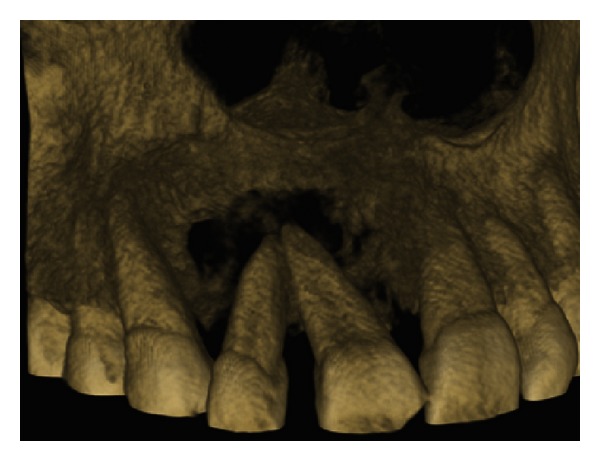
Reconstructed 3D CBCT image.
